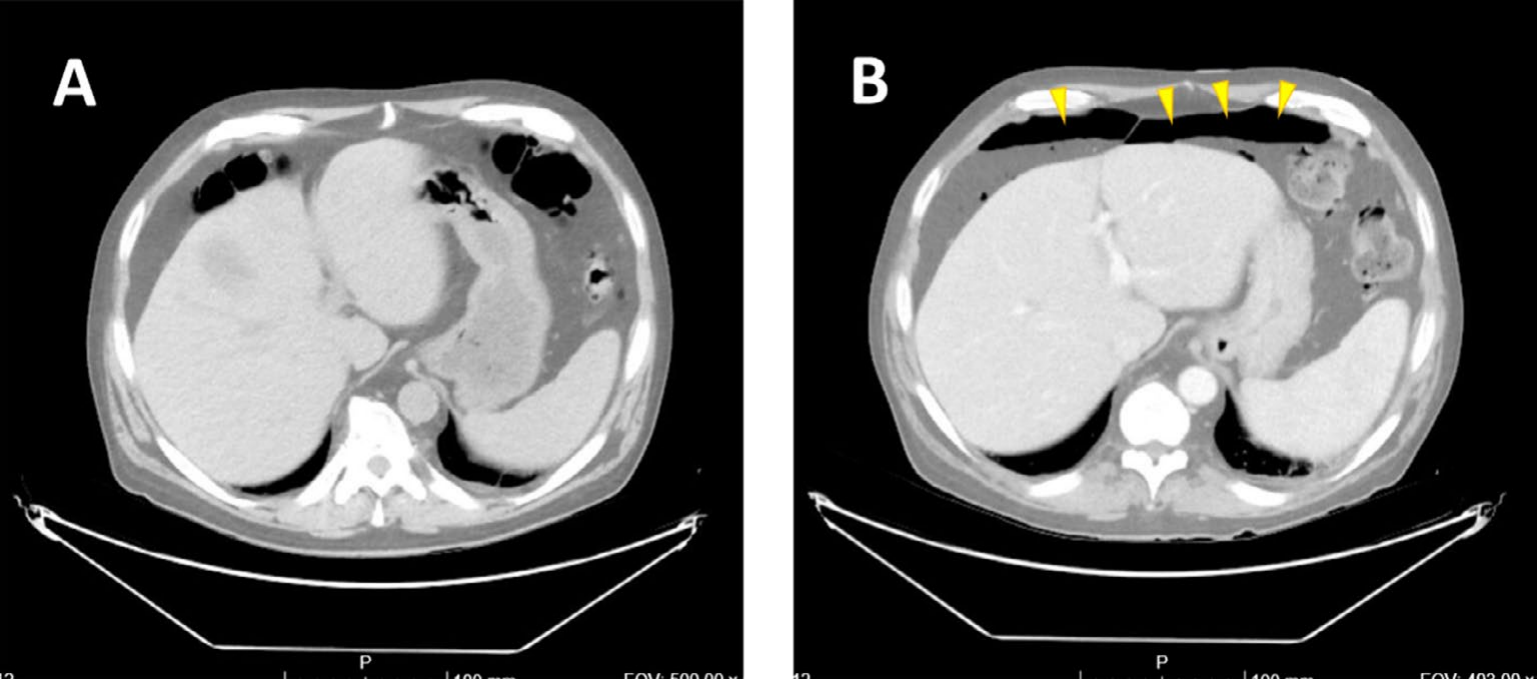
Comparison between urgent contrast‐enhanced abdominal CT scans performed during cycle 2 (A) and cycle 4 (B). The yellow arrows in B indicate free air in the abdomen, consistent with intestinal perforation.
